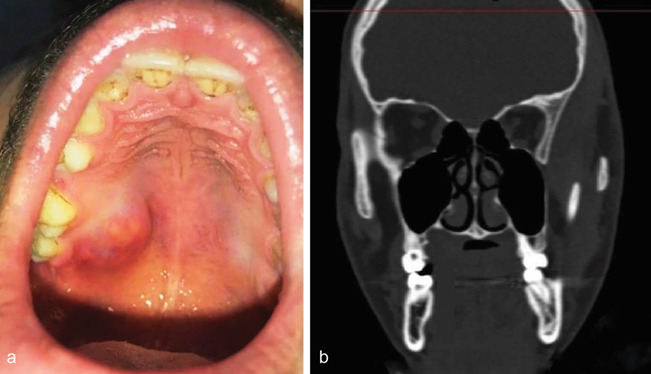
a: Clinical picture of the lesion, b: CT scan of the patient
